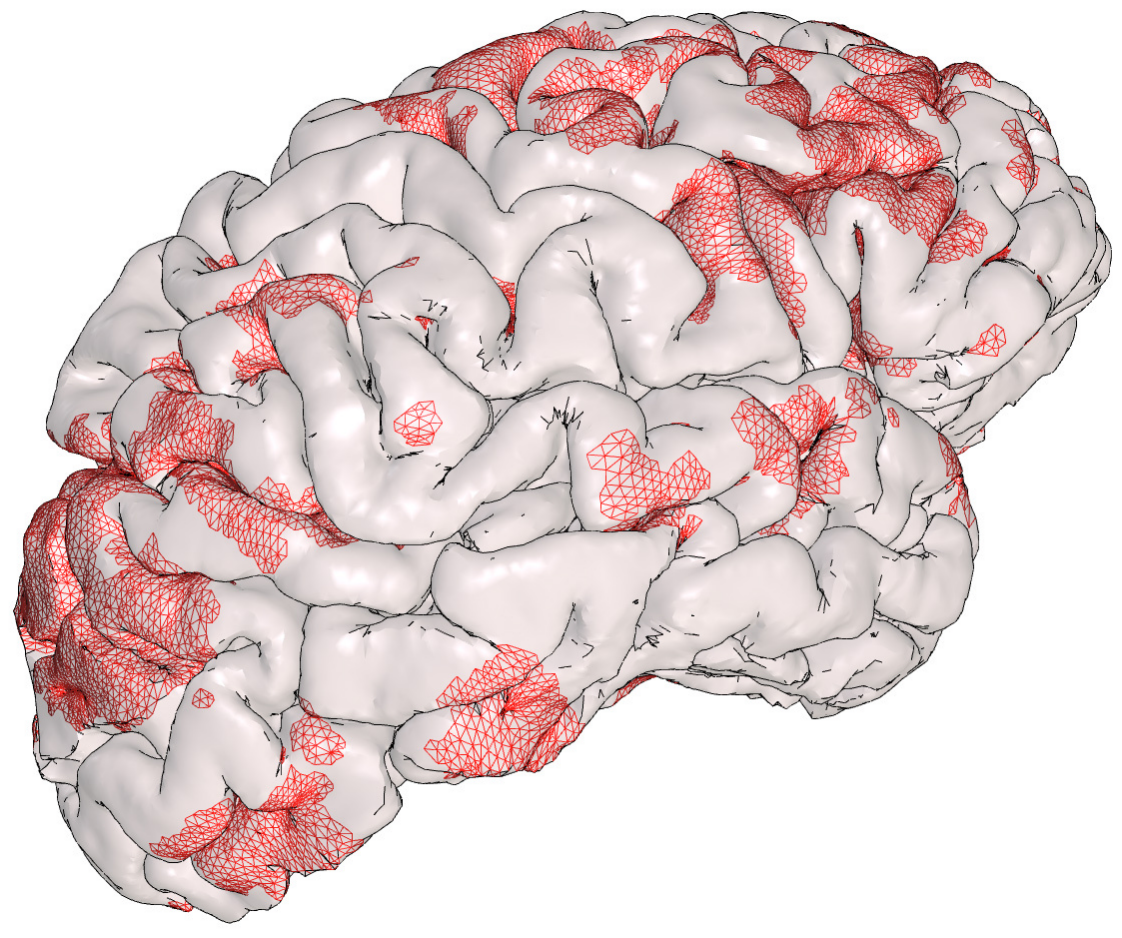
Surface rendering of the outer cerebral cortex (pial surface), and optional overlay of correlated functional activations corresponding to selected, recognised rest state networks (accessed via the “Views” menu when reading File S2). Functional MRI data set courtesy Govinda Poudel; cortical surface extraction by FreeSurfer (http://surfer.nmr.mgh.harvard.edu/).
